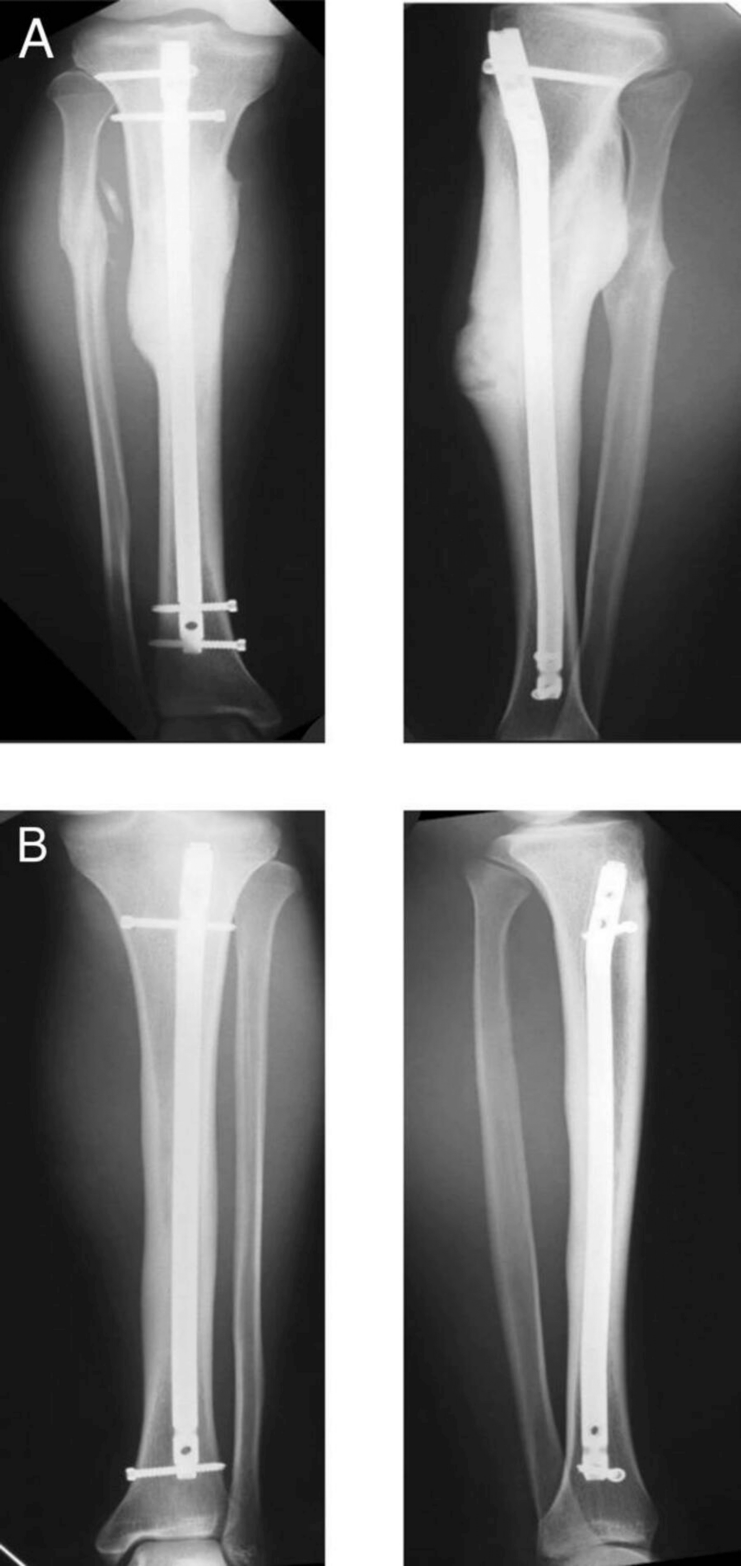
Radiographs demonstrate intramedullary nailing for the total and partial stabilization of tibial fractures
